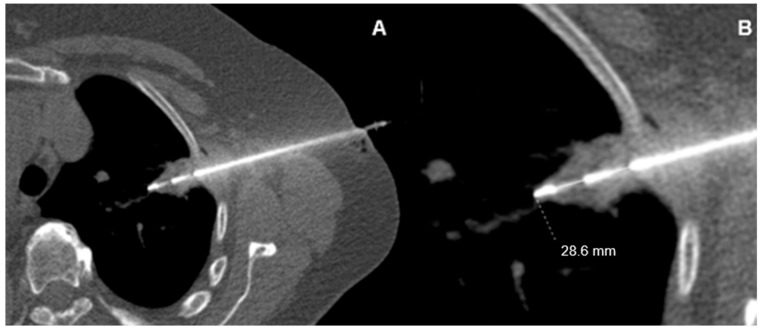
CT-guided lung biopsy in a pleura-adherent lesion. (A) Axial CT image showing a pleura-adherent lesion during biopsy. (B) Although the lesion contacts the pleura (0 mm distance), the needle penetrates 28.6 mm into the lung. This deep positioning ensures the biopsy cylinder is fully intrapulmonary, avoiding a punching defect in the pleura and minimizing pneumothorax risk.
